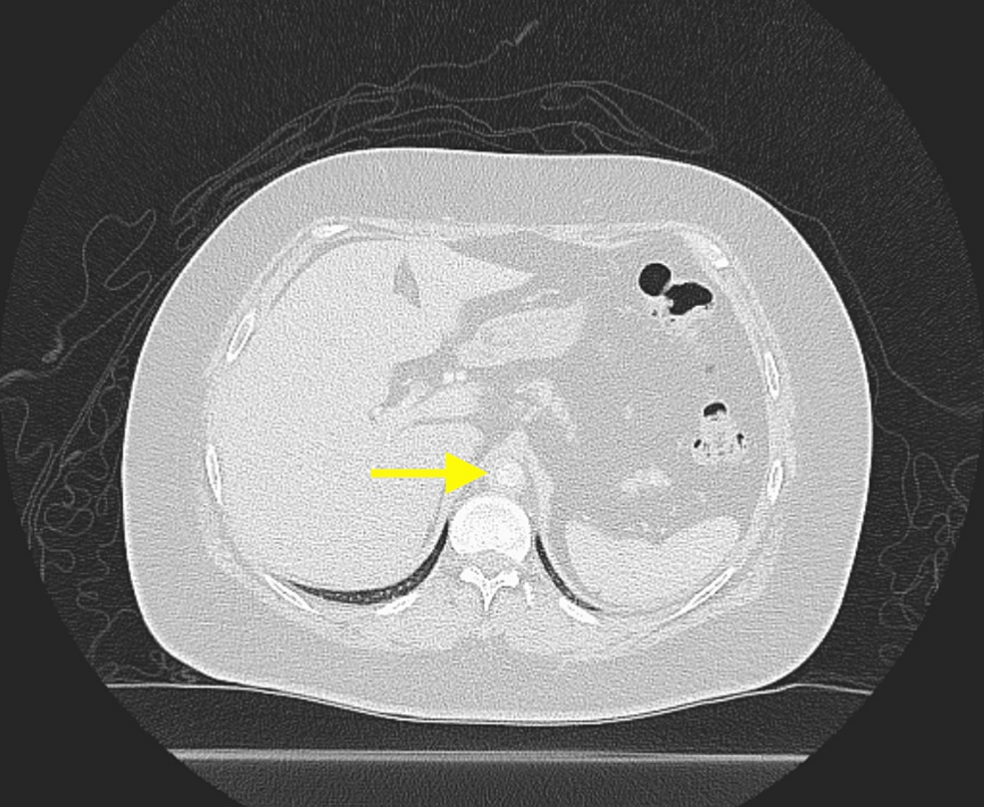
CT imaging of the abdomen and pelvis with contrast. The yellow arrow is pointing toward an extensive circumferential wall thickening along the ascending thoracic aorta, suggesting an inflammatory vasculitis, likely Takayasu arteritis.
